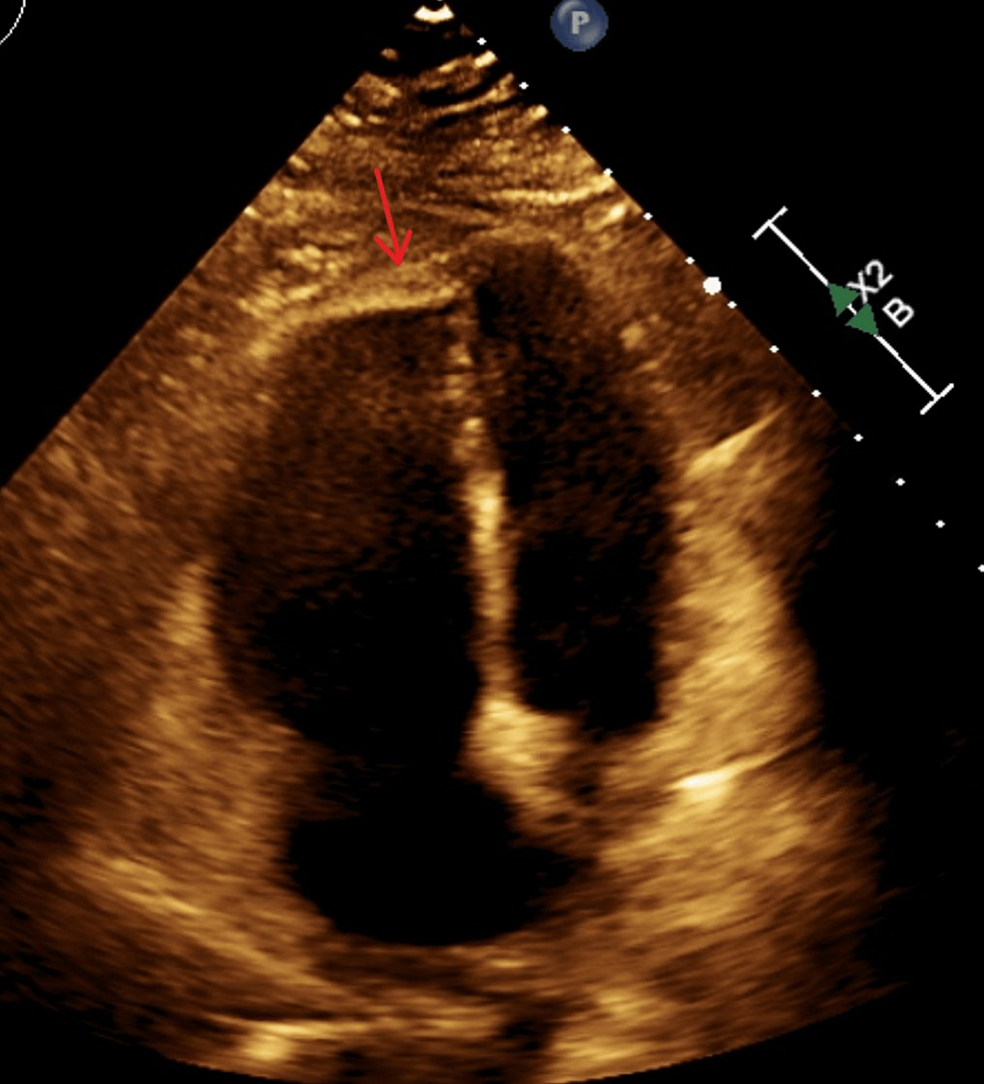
Echocardiography in a 4-chamber view shows a grossly dilated RV and a normal-sized LV. The arrow is depicting McConnell’s sign, in which the right ventricular apex moves inward with contraction, whereas the right ventricular free wall moves outward with contraction due to akinesis. RV: right ventricle; LV: left ventricle
